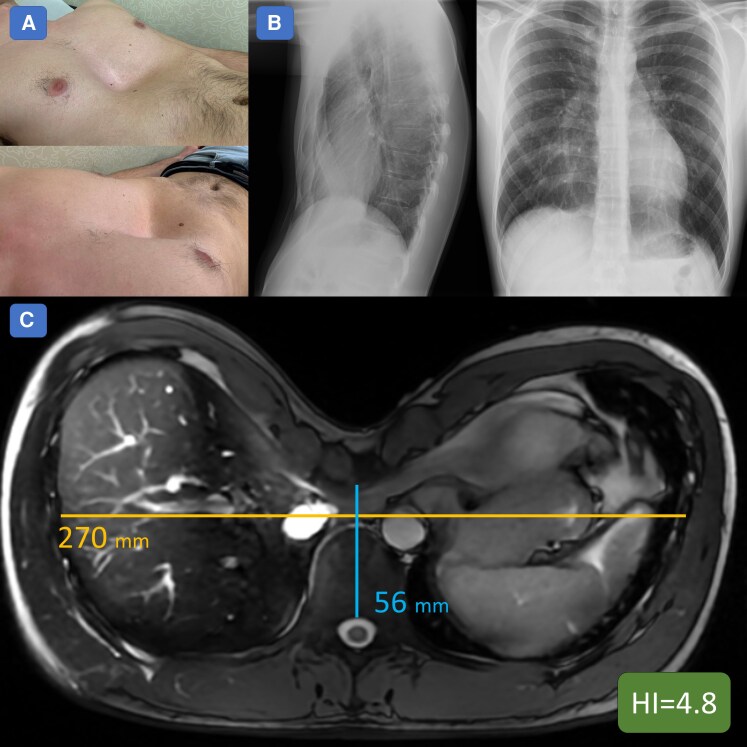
PEX anatomical and radiological findings. Panel A: PEX is clearly visible on physical examination. Panel B: Chest X-ray already shows typical features of PEX, including leftward displacement of the heart, blurring of the right cardiac border, and altered rib orientation; Panel C: CMR was also used to calculate the HI, which in this case was compatible with a severe form of PEX (4.8). *Abbreviations*: PEX, Pectus Excavatum; CMR, Cardiovascular Magnetic Resonance; HI, Haller Index.
